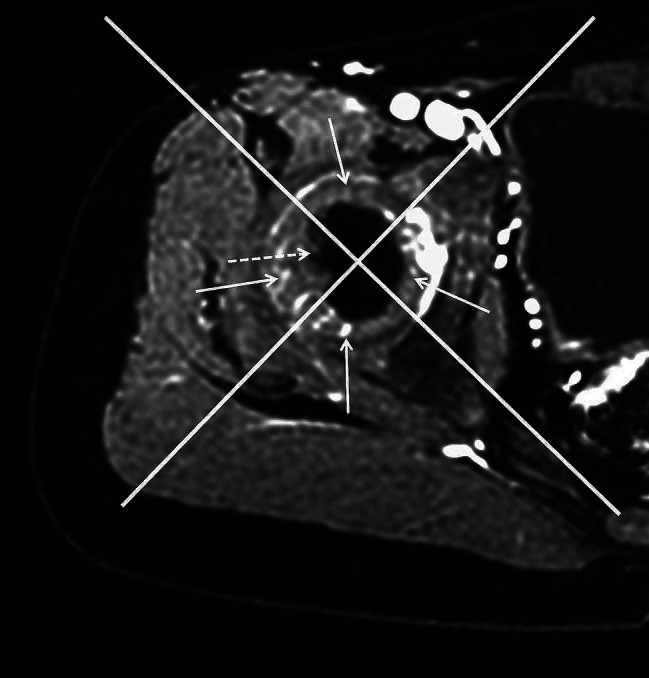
Enhanced MR image of the normal femoral head in a 4-year-old boy. The FH is categorized into four parts (medial, lateral, anterior, and posterior) by two perpendicular straight lines passing through the center of the FH. The 90° area directly in front is defined as the anterior part. The ossification center (dotted arrow) and cartilaginous (arrow) vessels are shown. MR = magnetic resonance, FH = femoral head
